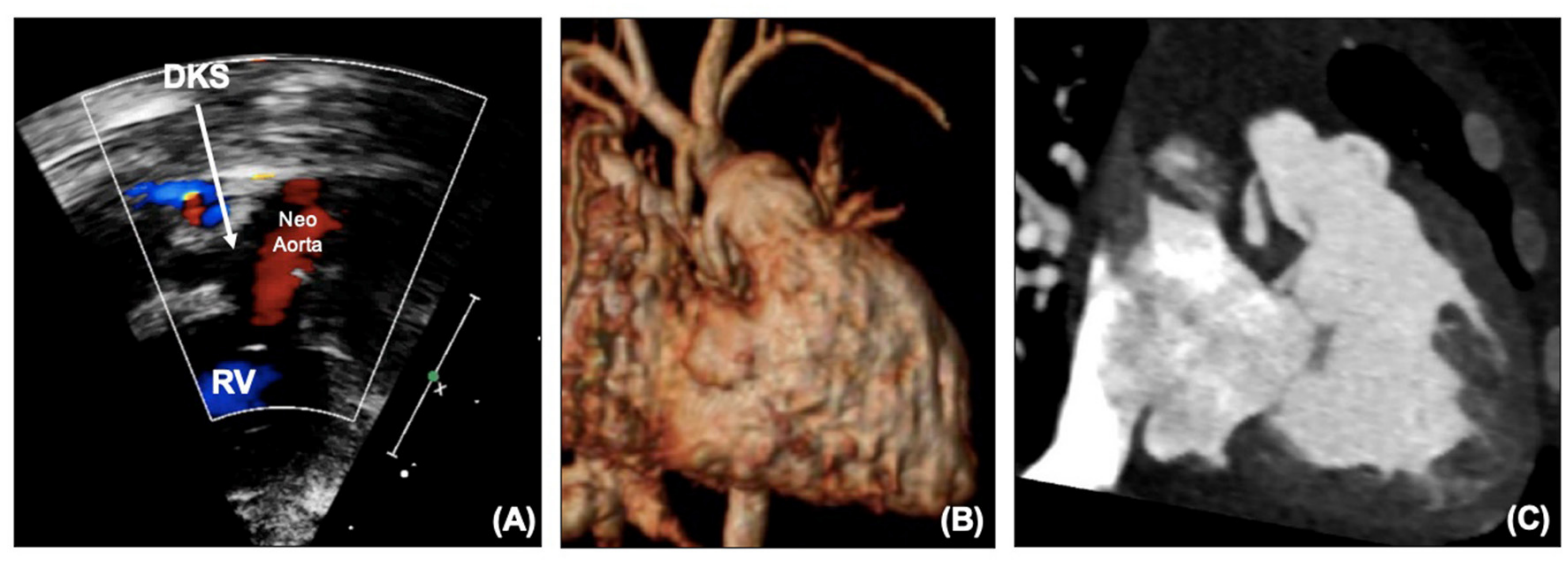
(A) DKS anastomosis on echocardiography. (B) DKS anastomosis on MRI. (C) DKS narrowing on CT. DKS, Damus-Kaye-Stansel; RV, right ventricle.
